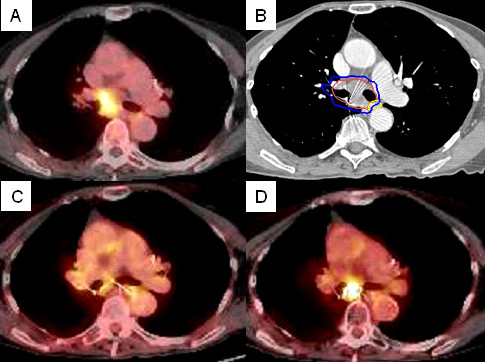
Right hilar tumor treatment planning PET/CT with a tumor SUV_max _of 7.0 (A), planned radiation dose distribution (B: the planning treatment volume is shown in red and the 30 Gy isodose line in blue), and PET/CT at 6, and 12 months post-treatment (C and D) show an initial decrease in SUV_max _to 2.5 followed by local recurrence (SUV_max _= 7.2).
